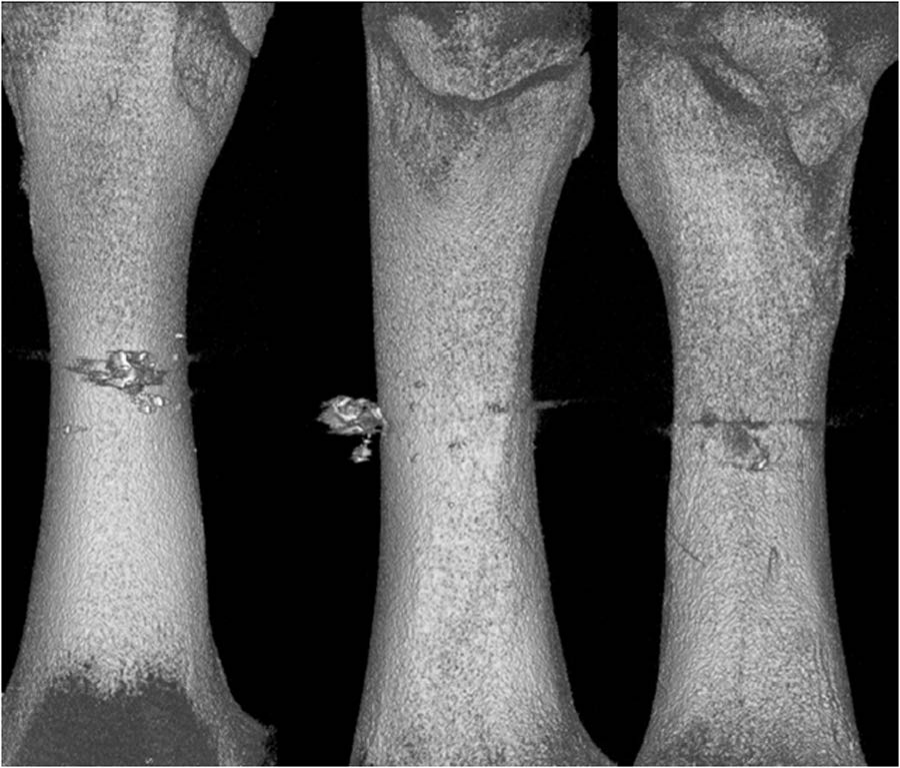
H&N Spitzkugel cal. 5.5 mm. 3D CT gunshot damage reconstruction. From the left: anterior, lateral and posterior shaft surfaces.
